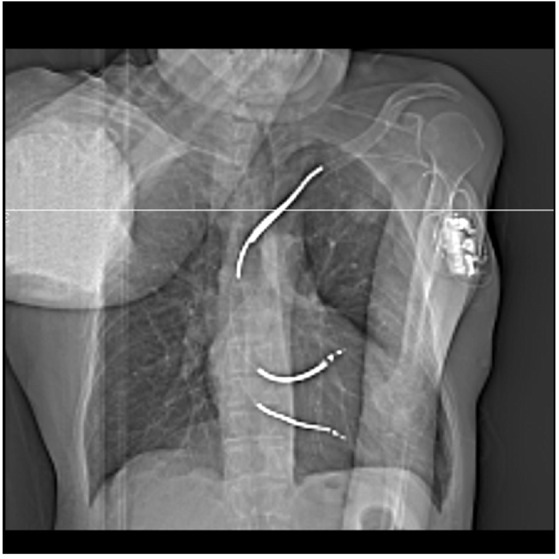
The lesion is seen on the CT topogram with a defibrillator in situ anteriorly and the scapula limiting access posteriorly.
